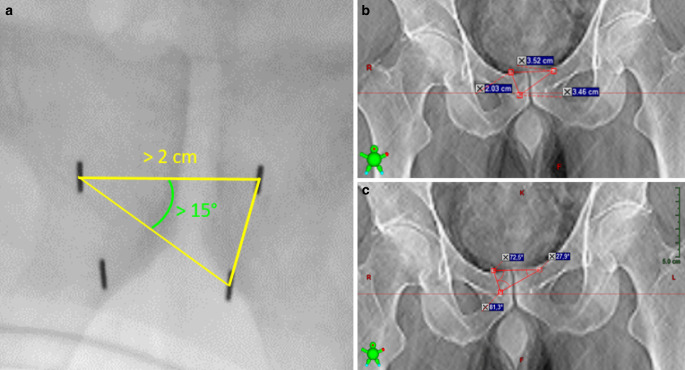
Triangulation over fiducial markers. a Connecting the center of mass of at least three fiducials yields a triangle whose side lengths and angles within the triangle shall exceed 2 cm and 15°, respectively, enabling the tracking system of the Cyberknife® (Accuray® Inc., Madison, WI, USA) to safely tell them apart and be able to calculate the uncertainty. Measurement of interfiducial distances (b) and angles (c) in a digitally reconstructed radiograph obtained from the planning CT
